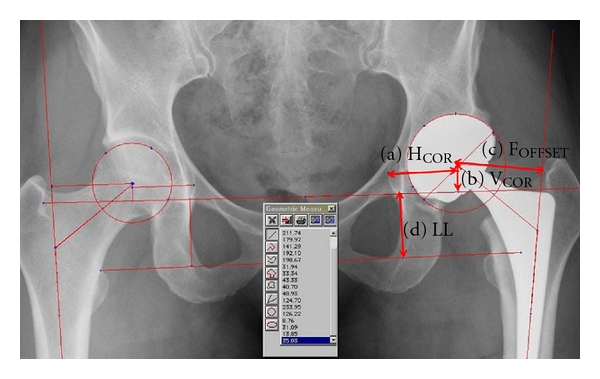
Radiographic measurement. (a) horizontal center of rotation (H_COR_), (b) vertical center of rotation (V_COR_), (c) femoral offset (F_OFFSET_), and (d) leg length (LL).
